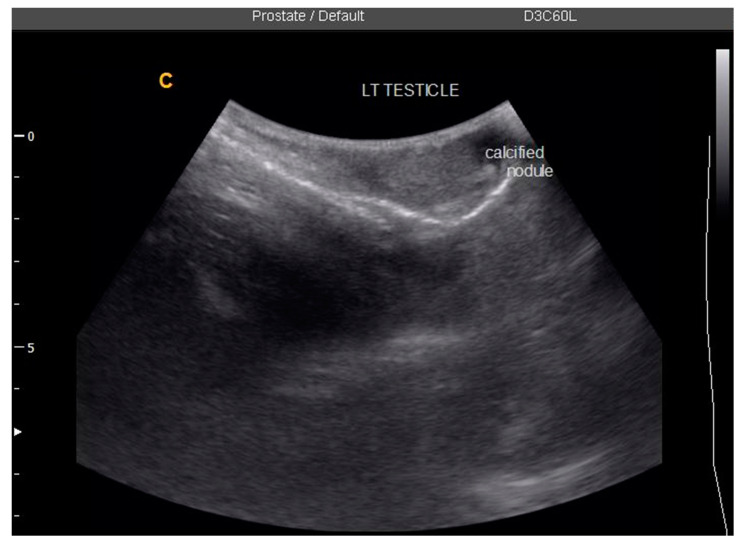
Ultrasonographic image of study participant with left testicular nodule at baseline.
